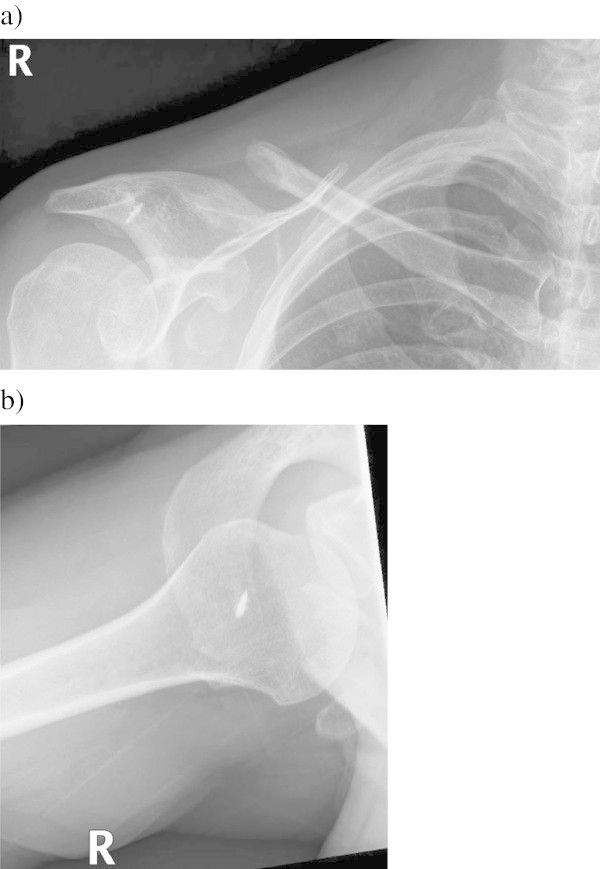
Anteroposterior (a) and axillary (b) radiographs of a patient who had surgery for chronic AC joint dislocation with autogenous semitendinosus and gracilis tendon grafts 4 years previously. Marked osteolysis has developed to the lateral clavicle. This patient also suffered a fracture of the coracoid process.
